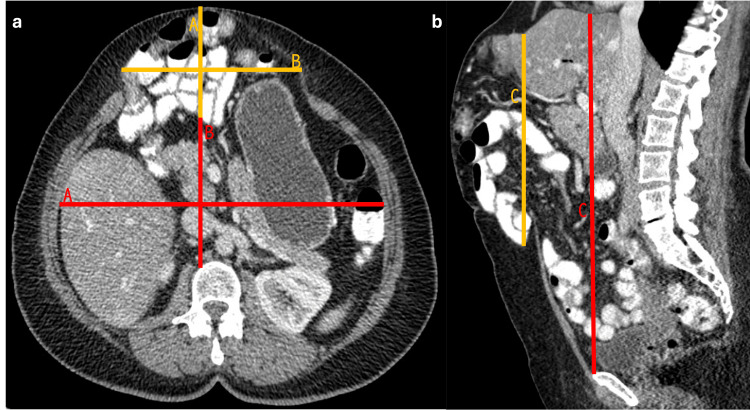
Axial and sagittal abdominal CT scan showing the Tanaka Method (a, b) Axial and sagittal CT scan showing the anteroposterior (A lines), transverse (B lines), and longitudinal (C lines) dimensions of the hernia sac and abdominal cavity to calculate volumes and then apply the Tanaka method. The figure was created by the authors.
